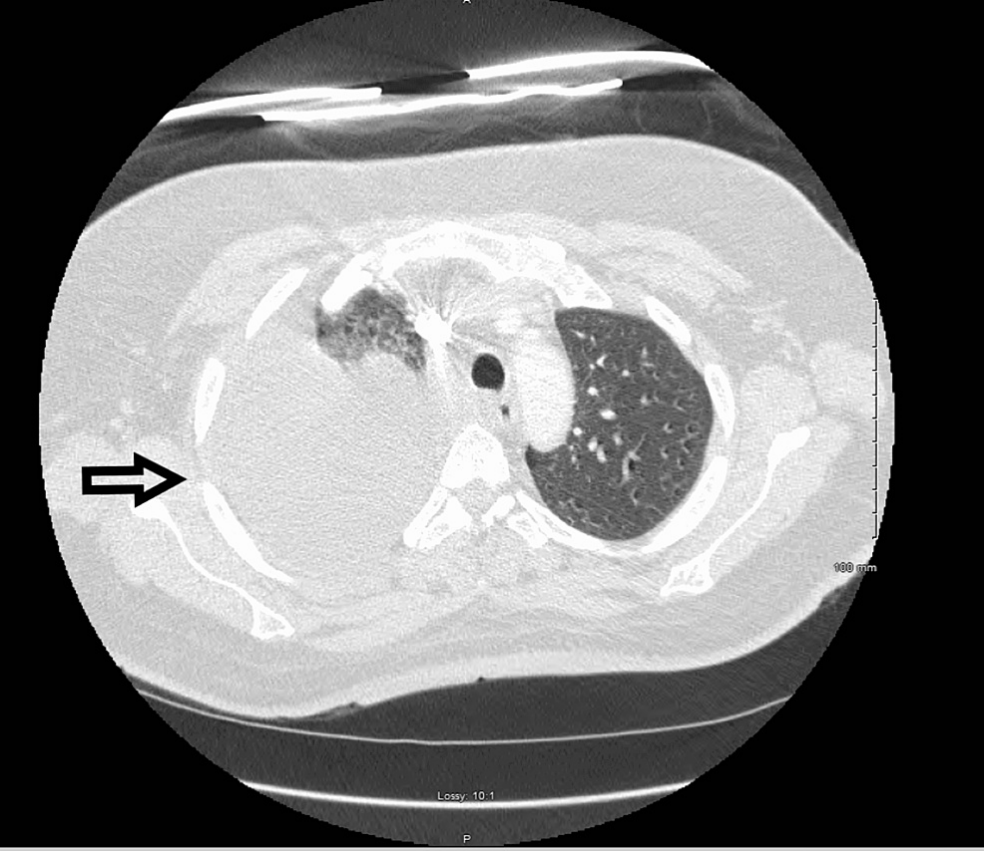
CT chest showing right-sided pleural effusion.
